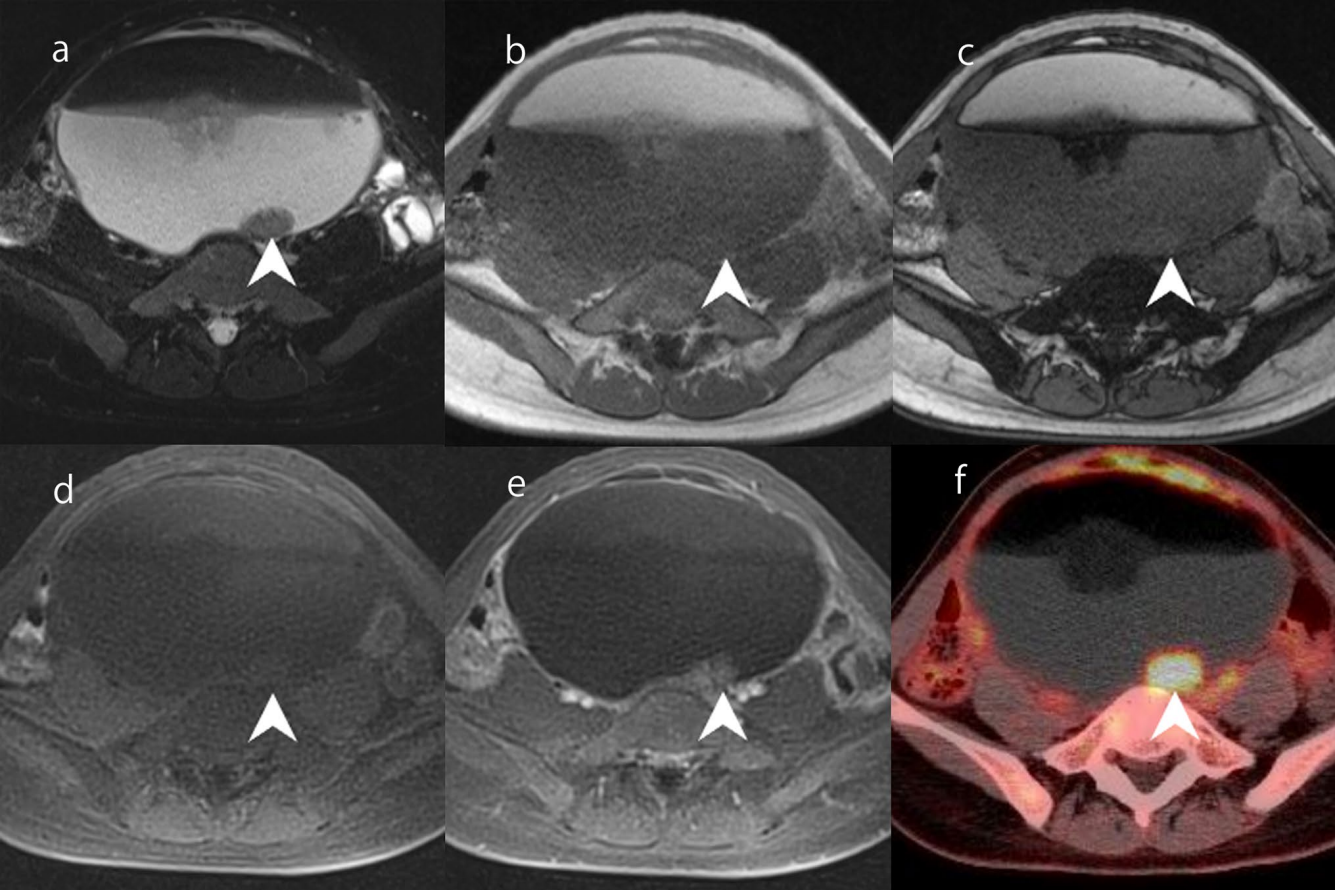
Malignant transformation of mature cystic teratoma in a woman in her 20 s. a FS-T2WI shows a cystic mass with an internal fluid–fluid level. A moderately hyperintense 2-cm mural nodule on the posterior wall of the mass (arrowhead) is observable on FS-T2WI. T1-weighted gradient-echo in-phase (b) and opposed-phase (c) imaging shows that the mural nodule has no fat or lipid content (arrowhead). d FS-T1WI shows lipid-rich fluid and a hypointense mural nodule (arrowhead). e Gd-enhanced FS-T1WI shows mild enhancement of the mural nodule (arrowhead). f 18F-FDG-PET/CT shows significant accumulation in the mural nodule (arrowhead). Surgery was performed and malignant transformation (squamous cell carcinoma) of mature cystic teratoma was diagnosed
